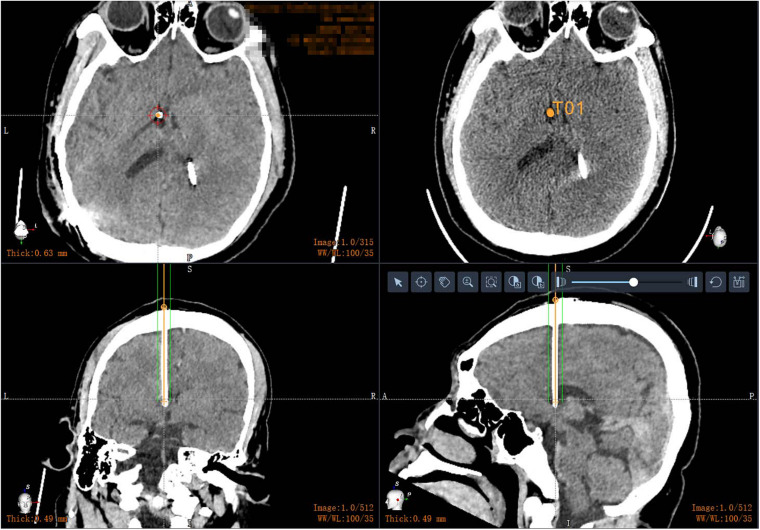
Postoperative CT in patients with small ventricles: robot-assisted drainage tube precision implantation.
